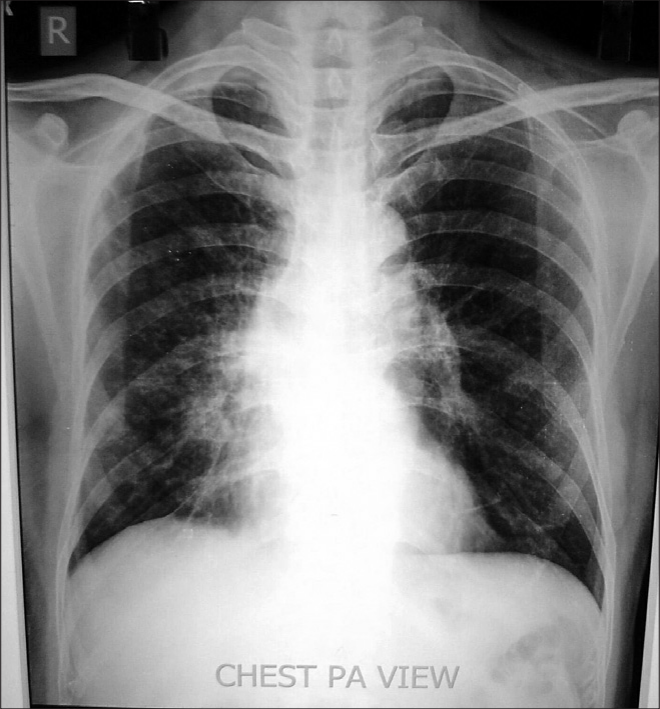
Chest X-ray reveals unfolding of aorta, hazy right parahilar and para cardiac area
